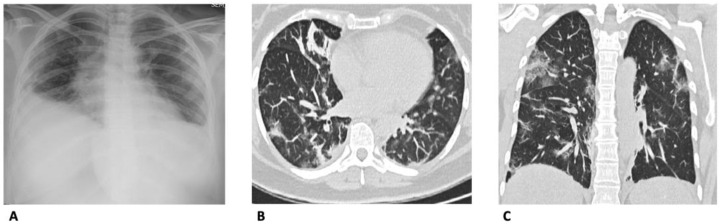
Discordant imaging findings between chest X-ray and CT in a patient with SARS-CoV-2-related pneumonia. Lesions suggestive for SARS-CoV-2-pneumonia were not evident on anteroposterior CXR (A). On CT [(B) in the axial and (C) coronal planes], however, multiple reticulations and GGOs are found in the subpleural areas of the lungs bilaterally.
